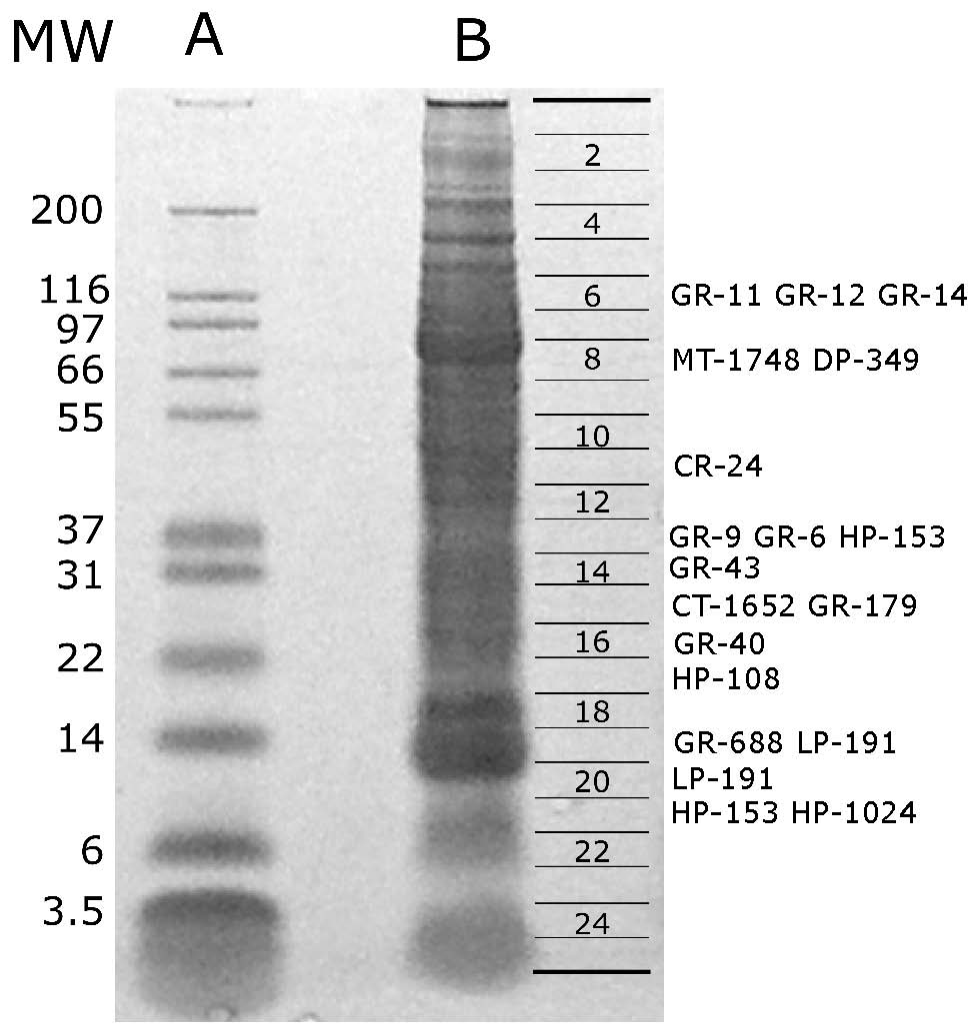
Sodium dodecyl sulfate polyacrylamide gel electrophoresis of salivary gland homogenate of *Amblyomma variegatum*. Lane A shows molecular mass markers, with their masses indicated in column MW. Lane B represents the tick sample. The grid at the right indicates how the gel was cut for tryptic digest of the peptides, and the two-letter-number code indicates the putative secreted proteins identified at the gel bands. The two-letter codes stand for: GR, glycine-rich protein; MT, metalloprotease; DP, dipeptidyl peptidase; CR, calreticulin; HP, hypothetical protein; CT, cytotoxin-like; and LP, lipocalin. The numbers indicate the Amb_var protein number available in Supplemental Table S2.
